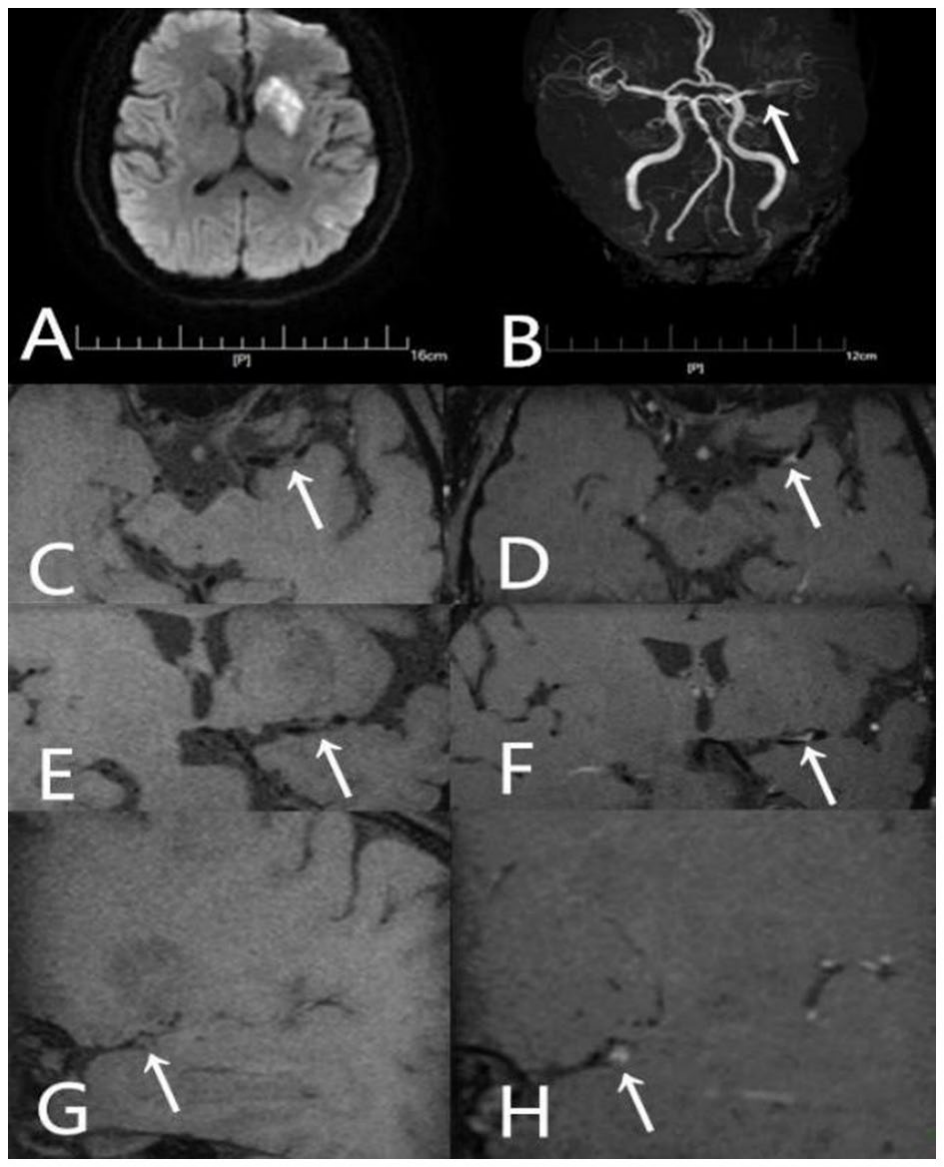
A 54-year-old male presented with aphasia and right-sided hemiparesis of one day’s duration. He had not been taking any statin medication prior to the onset. (A) DWI shows high signal intensity lesions in the left fronto-parieto-insular region and basal ganglia. (B) TOF-MRA demonstrates severe stenosis in the left middle cerebral artery (arrow). (C) Axial T1-weighted pre-contrast image shows the plaque (arrow). (D) Axial T1-weighted post-contrast image demonstrates eccentric wall thickening of the plaque (arrow) with enhancement. (E) Coronal T1-weighted pre-contrast image shows the plaque (arrow). (F) Coronal T1-weighted post-contrast image demonstrates eccentric wall thickening of the plaque (arrow) with enhancement. (G) Sagittal T1-weighted pre-contrast image shows the plaque (arrow). (H) Sagittal T1-weighted post-contrast image demonstrates eccentric wall thickening of the plaque (arrow) with enhancement.
